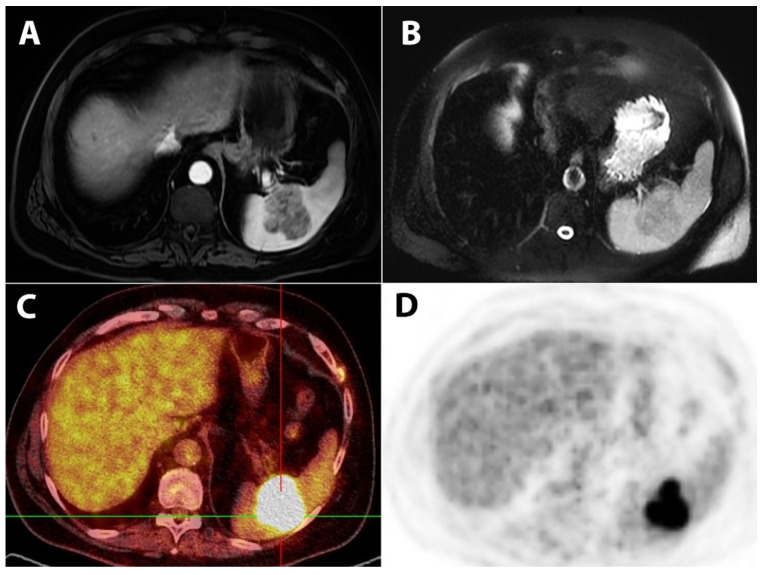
MRI T1-weighted FS image after gadolinium enhancement reveals a predominantly hypointense lobulated, relatively homogeneous splenic lesion (A) with discrete low signal intensity on T2W. (B) FDG-PET/CT (C) and FDG-PET axial image (D) show a splenic lesion with intensely increased FDG uptake.
